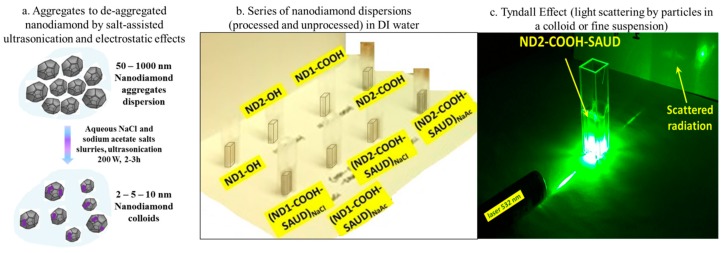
(a) Scheme of de-aggregation of nanodiamond following salt-assisted ultrasonication. The salts used are sodium chloride (NaCl) and sodium acetate (CH_3_COONa); (b) The series of nanodiamond dispersions and (c) Tyndall effect—light scattering by a representative de-aggregated nanodiamond (ND2-COOH-SAUD) colloid.
